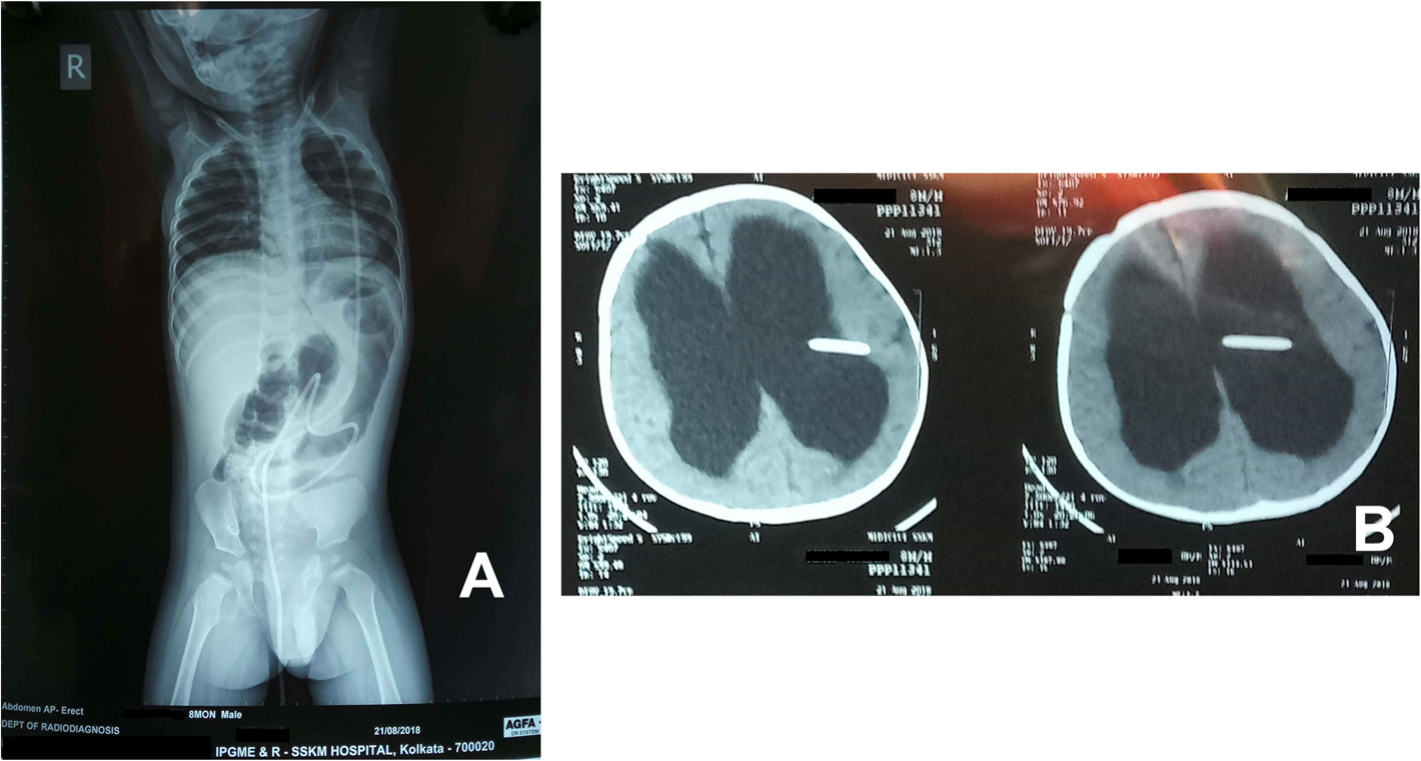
a Digital X-ray of whole abdomen postero-anterior view in erect posture shows expulsion of radio-opaque distal catheter tip through the anus into the exterior without any pneumoperitoneum. b NCCT scan shows the cranial catheter tip in the lateral ventricle of the brain
